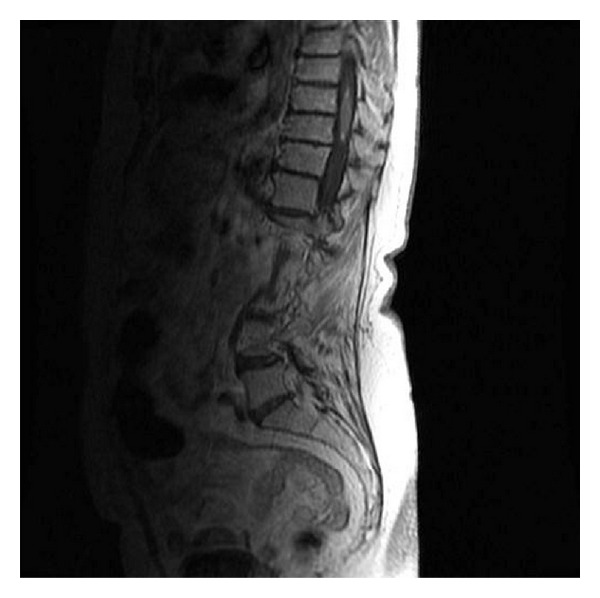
Thoracic MRI demonstrated a solitary intramedullary lesion at thoracic 9-10 level with homogeneous contrast enhancement in sagittal reconstruction.
